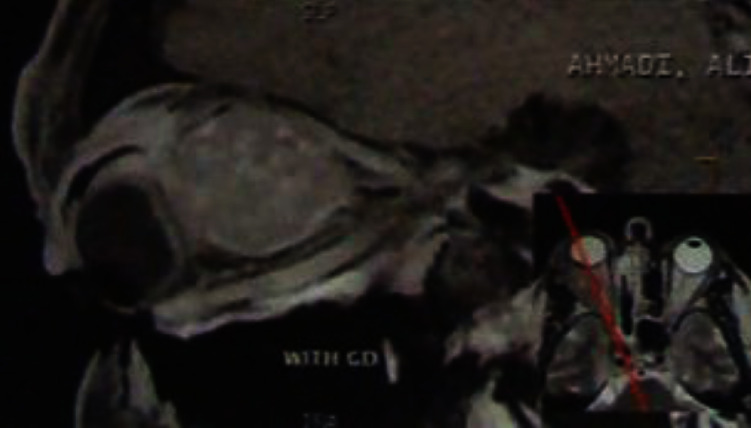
Sagittal MRI scan showing orbital mass with lateral rectus involvement.
